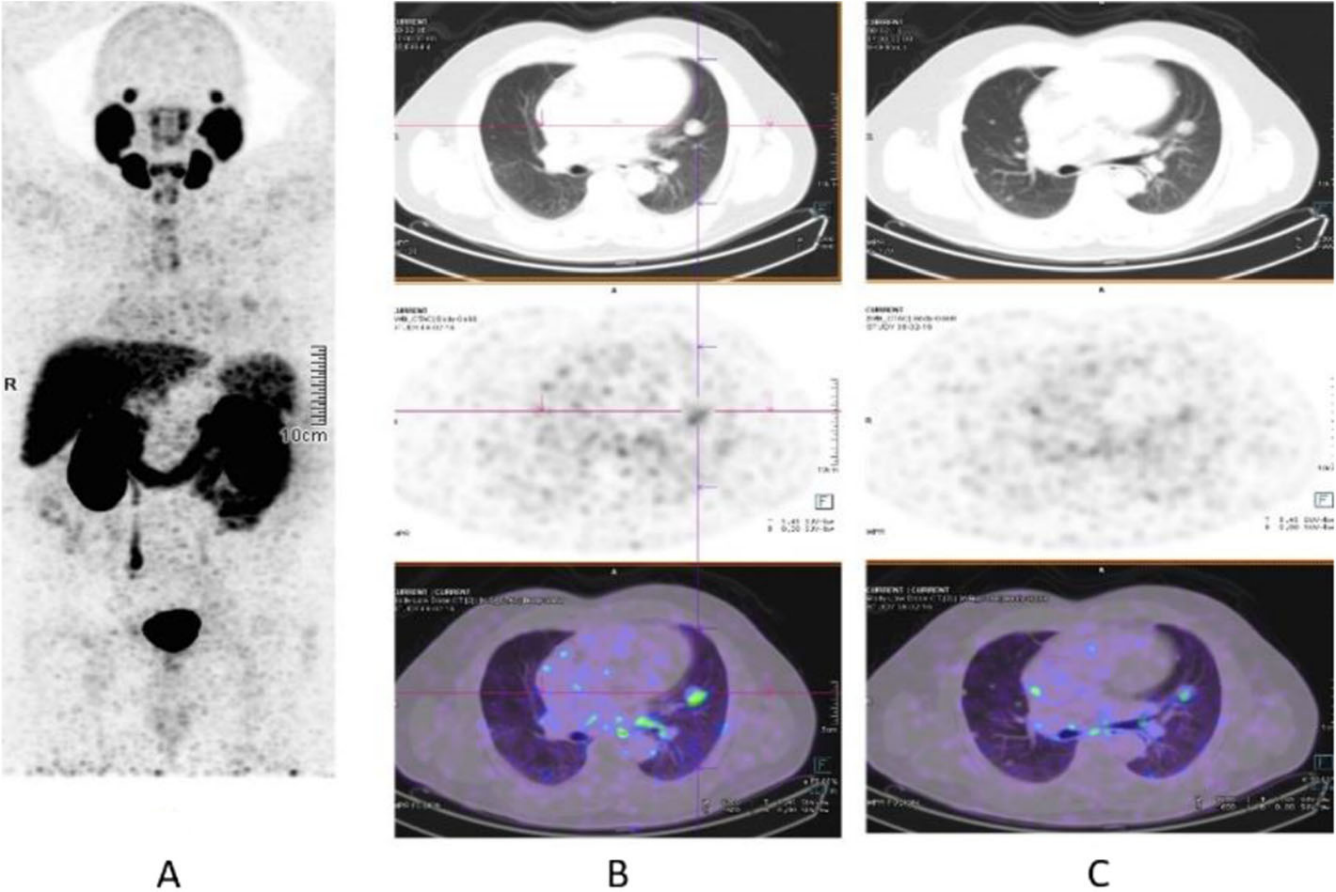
^68^Ga-PSMA, prostate cancer, biochemical recurrence (BCR): pulmonary metastasis. *Clinical history*: 66 y.o. man with prostate cancer (Gleason score 4 + 3, pT3N0Mx), radical prostatectomy, and adjuvant RT. Three years later PSA = 1.62 ng/ml, PSAdt < 6 months. Bone scan, abdominal CT, and pelvic MRI were all negative. *PET/CT findings*: mild tracer uptake in multiple pulmonary nodules visualised on CT, the largest nodule is 19 mm, in the lingula. a MIP; b, c CT, PET, and fused images
